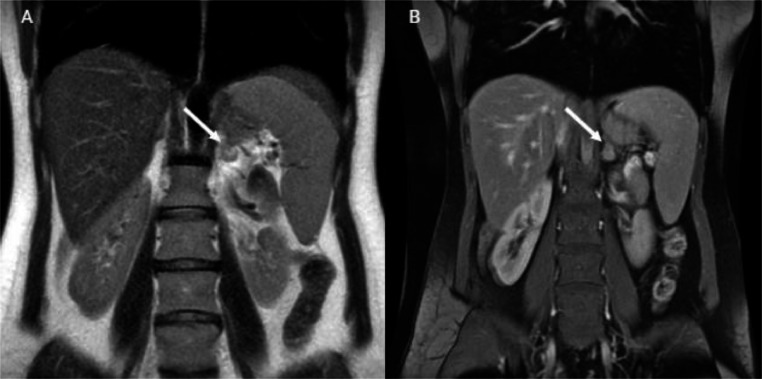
Coronal view of contrast-enhanced magnetic resonance imaging. (A) T2-weighted image revealing an iso-signal lesion in left adrenal gland (arrow). (B) T1-weighted image demonstrating a moderately enhanced lesion in the left adrenal gland (arrow).
